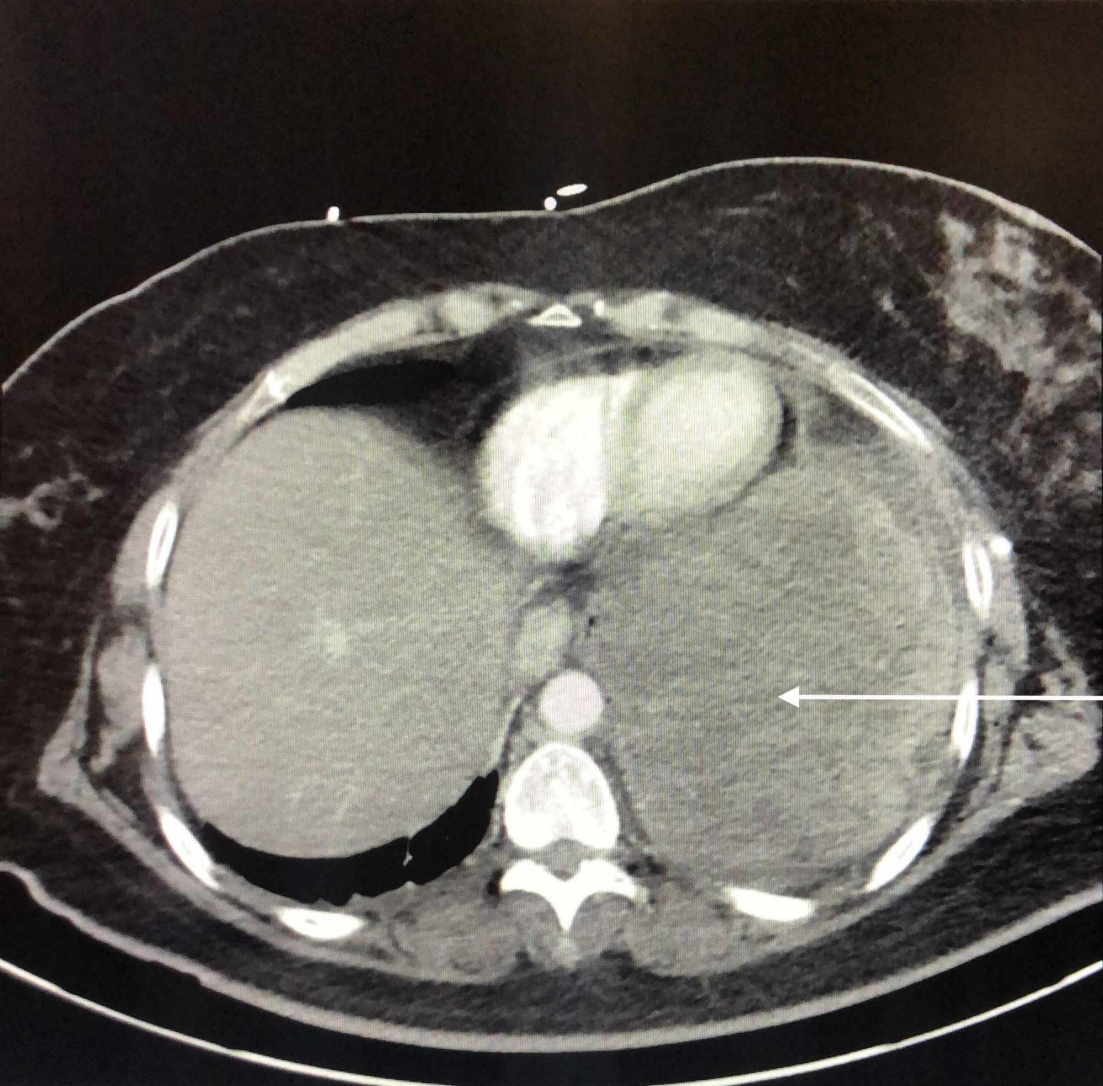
Chest CT shows a large heterogeneous left lower lobe mass with significant attenuation of the medial left lower lobe airway. Findings are concerning for a neoplastic etiology. Additional multifocal ground-glass/nodular opacities throughout the left hemithorax could be secondary to multifocal infection or patchy areas of pulmonary edema. No suspicious right hemithorax nodules are appreciated.
